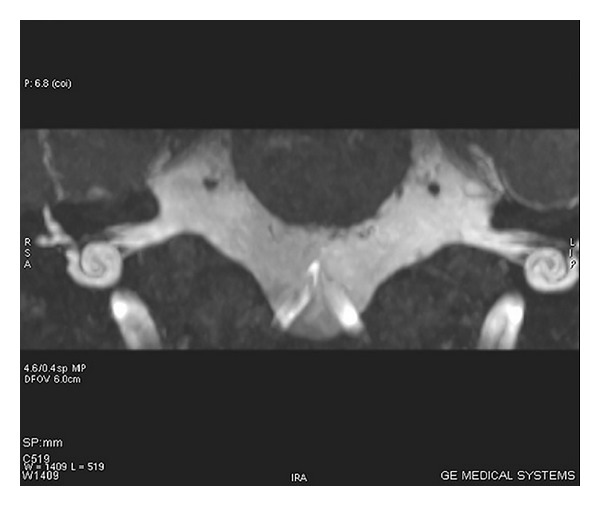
MRI scan of inner ear with axial section showing cochlear anomaly in which cochlea shows 1 and 1/2 turns.
